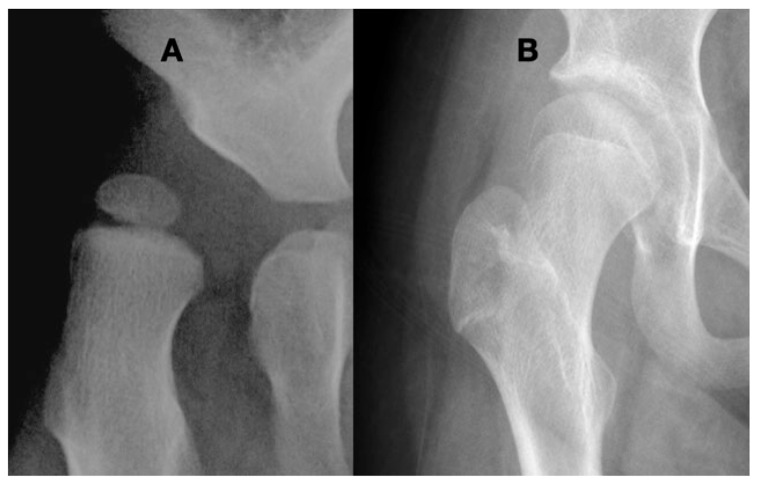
A 2.5-year-old girl was presented at the stage where she was just able to stand in her walker. This is her first screening radiograph (A) showing coxa valga, eccentric femoral epiphysis, severe subluxation, and acetabular dysplasia. Infantile hip ultrasounds were reported as normal. The etiology of this hip was felt to be due to her spasticity. Hip adductor lengthening was performed with immediate return to therapy and no splinting or casting. At the age of 14 years, she is at a Gross Motor Function Classification System level III and is walking with crutches, and her hip is well covered but still has a mild superior placement of the acetabulum as evidenced by the break in Shenton’s line (B). The only treatment for this hip was force balancing by a muscle-lengthening procedure, which was then enhanced by her increased walking ability as her motor development improved.
